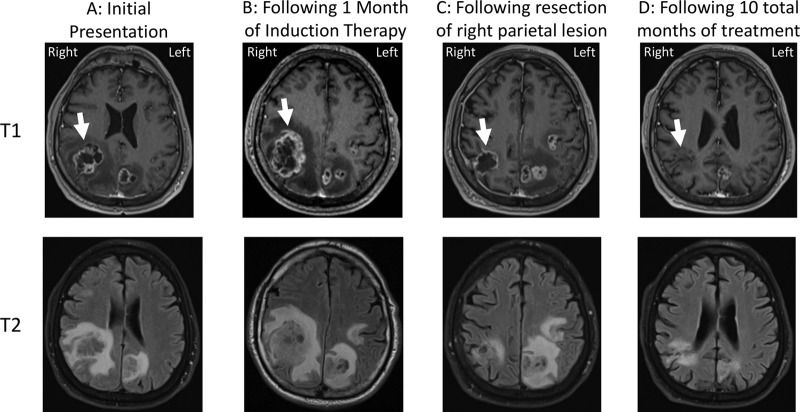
Changes in parietal lobe lesions on brain MRI over the course of treatment. The top panels represent post-gadolinium T1-weighted images, and the bottom panels represent post-gadolinium T2-weighted images. (A) MRI at initial presentation. Circumferential ring enhancement on the post-gadolinium T1-weighted axial image and increased signal surrounding the lesion on the axial T2-fluid attenuation inversion recovery (FLAIR) image represent marked vasogenic edema (arrow). The heterogeneously increased signal within the cavity of the lesions represents necrosis. (B) MRI following 1 month of induction therapy. Increased T2 signal within the lesion cavity on the FLAIR sequence and increased surrounding edema and the overall size of the right parietal lesion indicate an interval of worsening with likely progressive necrosis of brain tissue (arrow). A leftward midline shift was noted. (C) MRI following resection of the right parietal lesion. Note improvement in the edema surrounding the lesion on the FLAIR sequence and an interval of decrease in the size of the right parietal lesion (arrow). (D) Subsequent MRI imaging after a total of 10 months of treatment. Improved T2/FLAIR signal abnormality with a decrease in the size of the resection cavity (arrow) as well as decrease in the size of the other visible lesions was observed.
